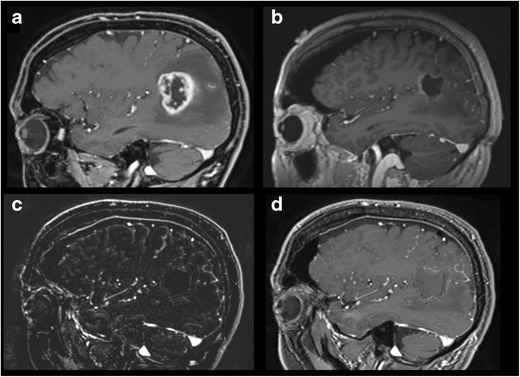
Pre-, intra-, and postoperative MRI of a patient harboring a high-grade glioma. a Pre-, b intra-, and c, d postoperative contrast-enhanced T1-weighted MRI of a patient harboring a glioblastoma. c demonstrates the subtraction imaging after Gd application. Postoperative MRI was performed within 48 h after surgery. Intraoperative imaging with iMRI, which demonstrated what appears to be a remnant rostral of the tumor cavity, was, moreover, revealed as Gd-leakage. Biopsy of this region demonstrated no tumor tissue, and Gd-enhanced postoperative MRI and subtraction imaging did not confirm tumor residual (c, d) (Courtesy of Dr. Ricardo Diez-Valle, Department of Neurosurgery, Clinica Universidad de Navarra, Navarre, Spain)
